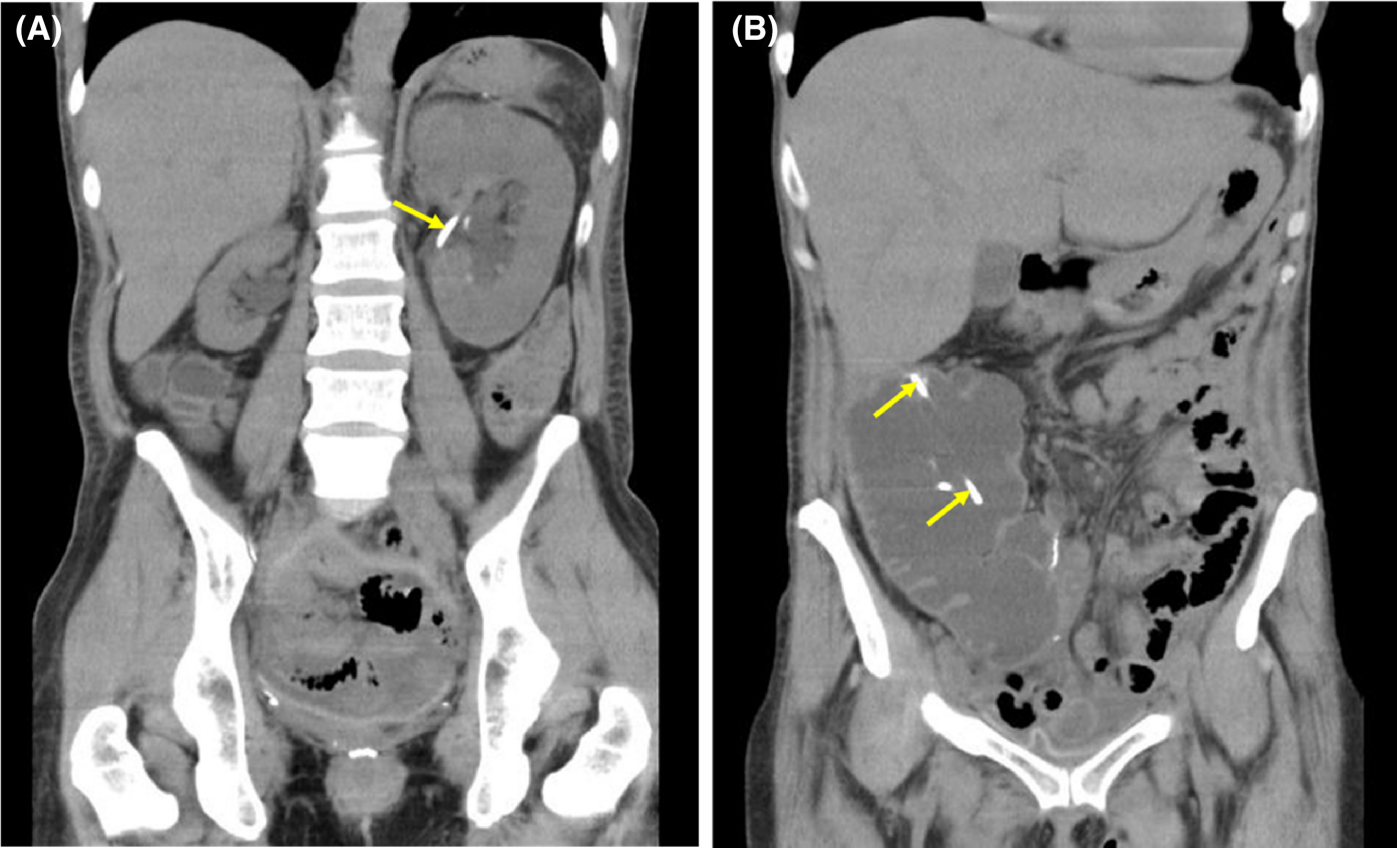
Plain computed tomography on the initial consultation. Bilateral mild hydronephrosis, right renal atrophy (A), and marked dilation of a urinary pouch (B) are observed. A ureteral stent (arrows) had been placed.
